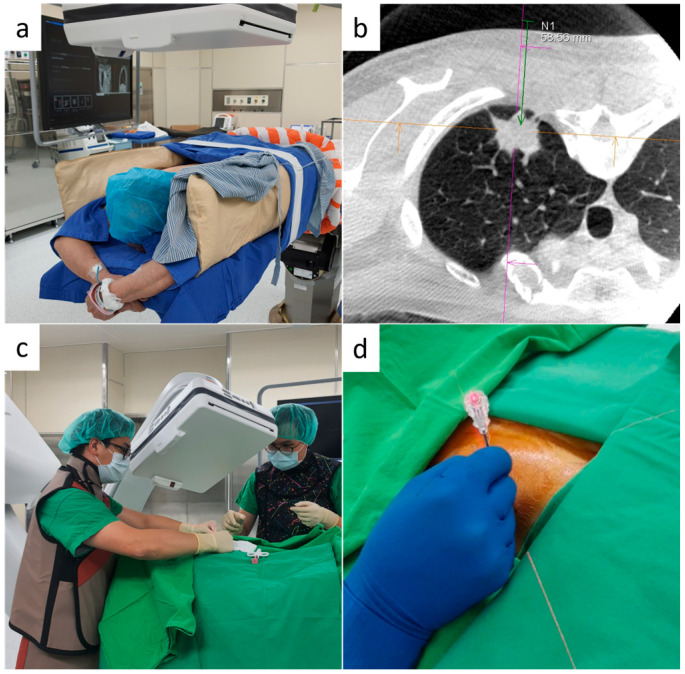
Patient preparation and settings for the iGuide biopsy procedure. (a) The patient was fixed in the prone position using a vacuum bean bag. (b) The lesions in the posterior region are visible in the CBCT images acquired in the same position (iGuide toolbox for lesion segmentation). (c) Interventional pulmonologists use the flat-panel detector of the C-arm to ensure the correct positioning of the laser beam. (d) The laser beam was projected onto the patient’s skin to guide the needle entry point. The needle was inserted into the chest wall after confirming that the centre of the laser cross was aligned with the outer tip of the needle.
